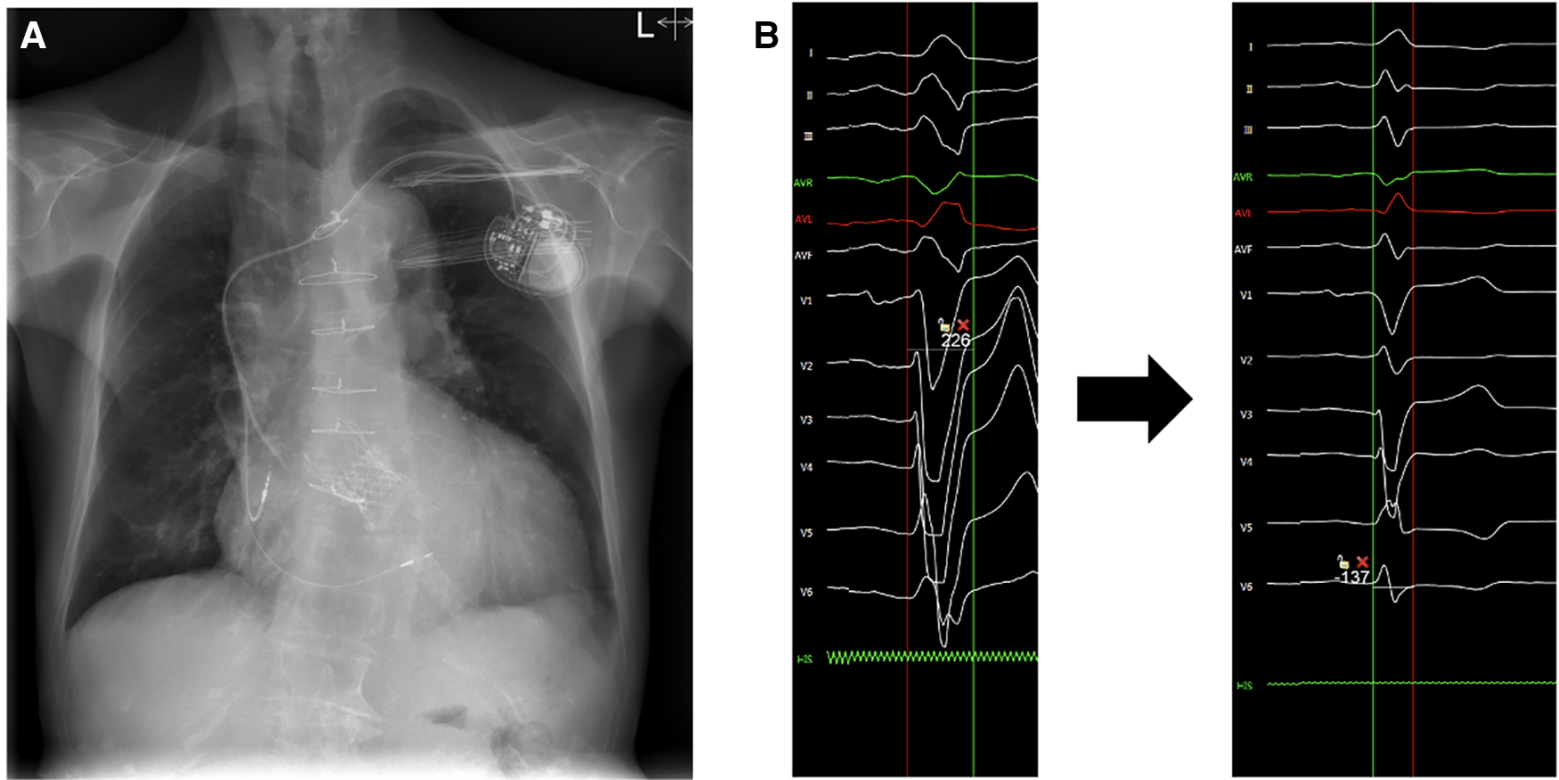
(A) Postoperative x-ray of the dual-chamber pacemaker with the atrial lead positioned in the right atrial appendage and the ventricular lead positioned transeptally for left bundle branch pacing (LBBP). (B) (Left) Initial QRS complex with left bundle branch block morphology. (Right) Final QRS duration after atrio- ventricular delay optimization.
